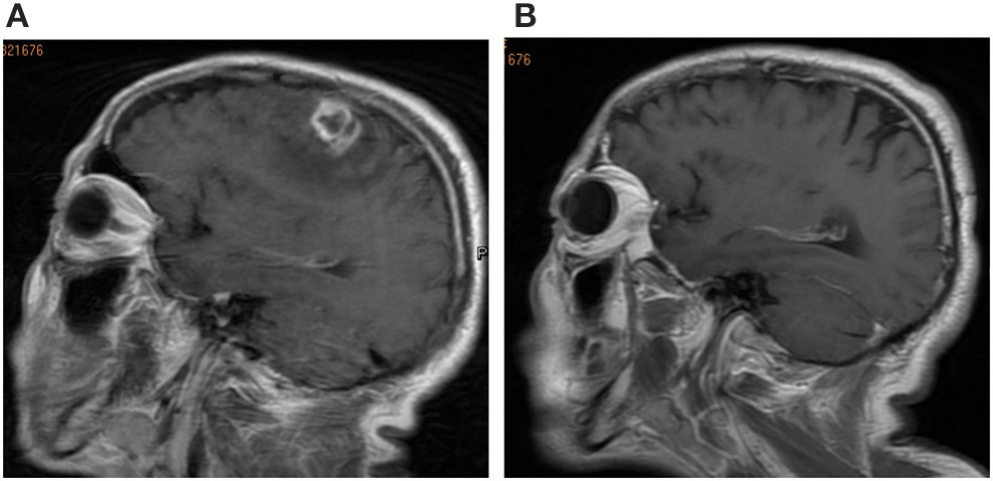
(A) Brain magnetic resonance imaging (MRI) revealed an irregular enhancing mass with central necrosis on the left parietal lobe. (B) Brain MRI image after surgery at the follow-up 3-mouth.
